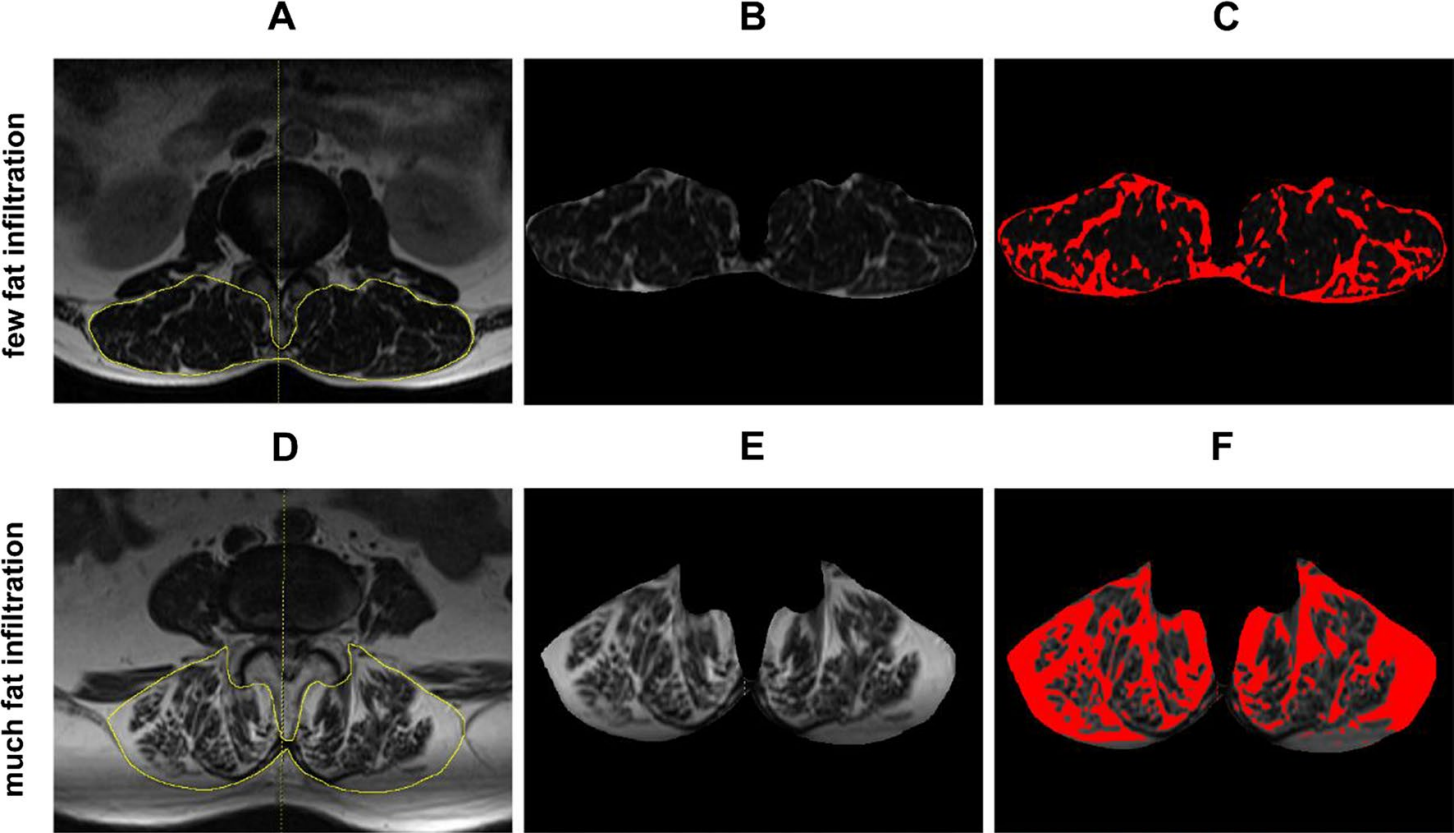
Cross section of the lumbar spine. T2WI MRI and calculation of the cross-sectional area of LPM and fat infiltration. A–C From the same patient with little fat infiltration; D–F From the same patient with much fat infiltration; B, E CSA of LPM on the same level; C, F CSA of fat tissue (red)
